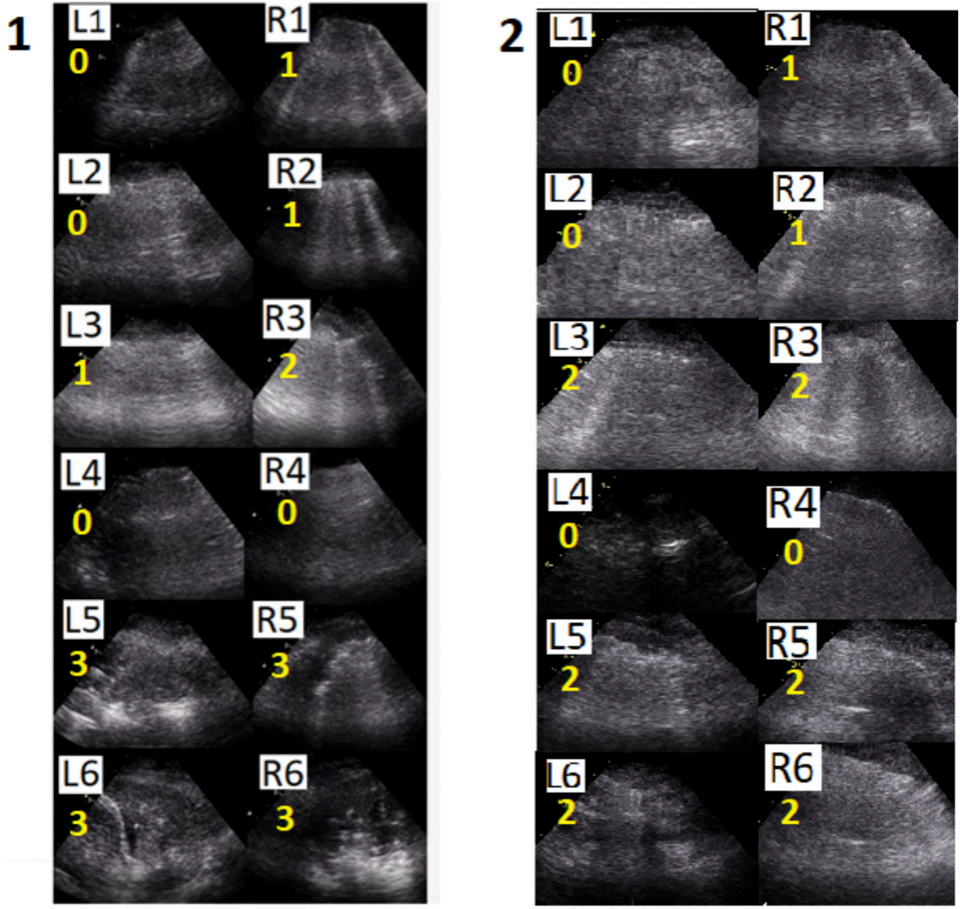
Lung ultrasound images for patient A, before (panel 1) and after the recruitment maneuver (panel 2). For each image, the corresponding score is presented (0–3). L = left hemithorax; R = right hemithorax; 1, 2 = anterior thoracic areas; 3, 4 = lateral thoracic areas; 5, 6 = posterior thoracic areas.
